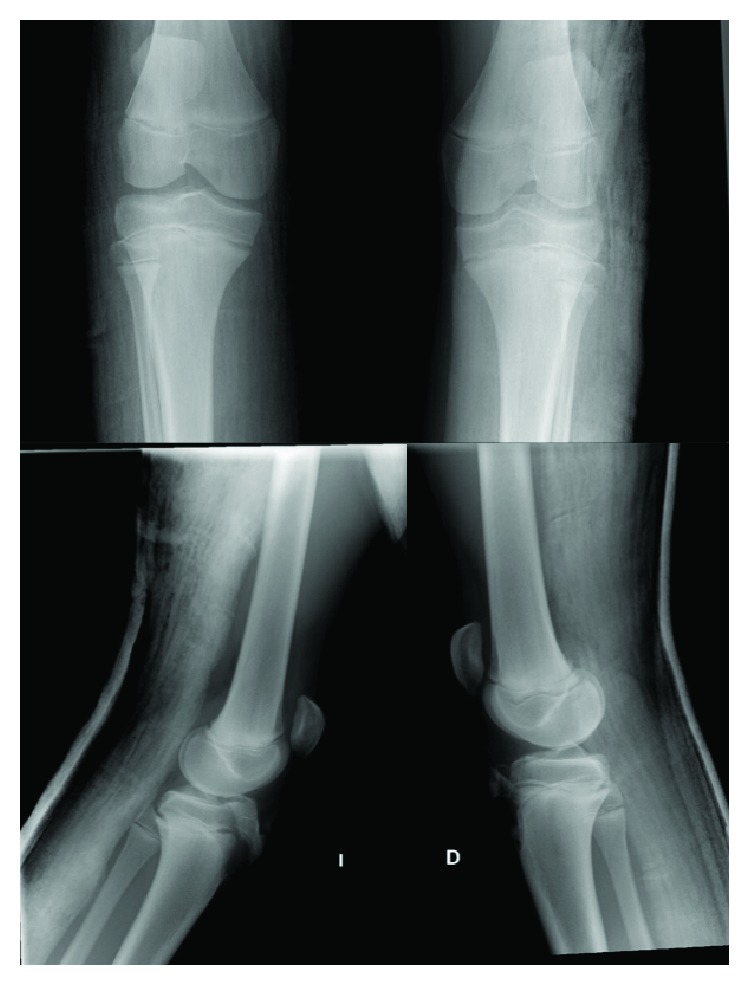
Plain radiographs of both knees. Right knee (D) showing an Ogden type IB fracture and the left one (I) type IIIA Ogden fracture.
